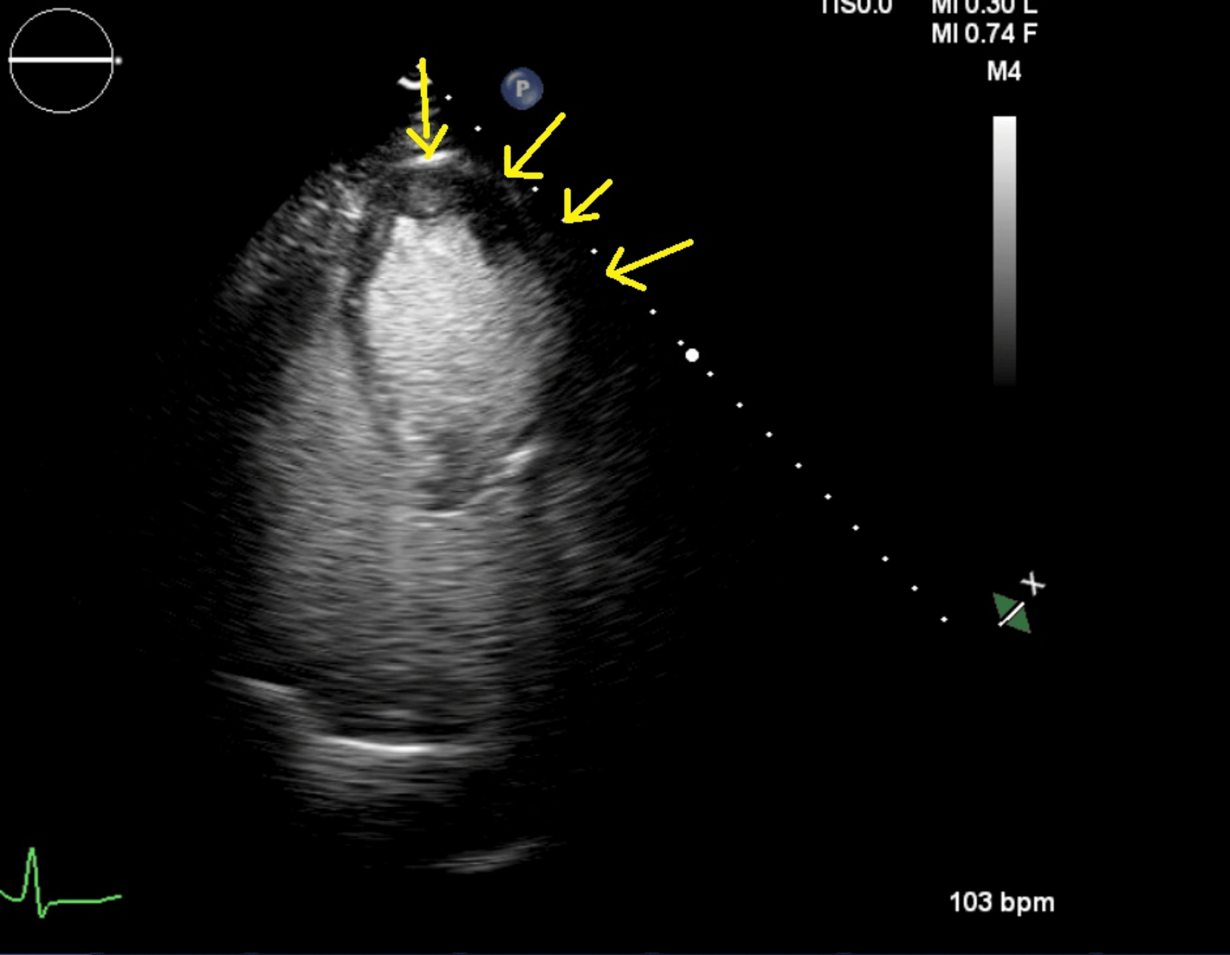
Transthoracic echocardiogram in systole demonstrating akinesis of the basal and midventricular segments with preserved apical contraction (marked by arrows)
